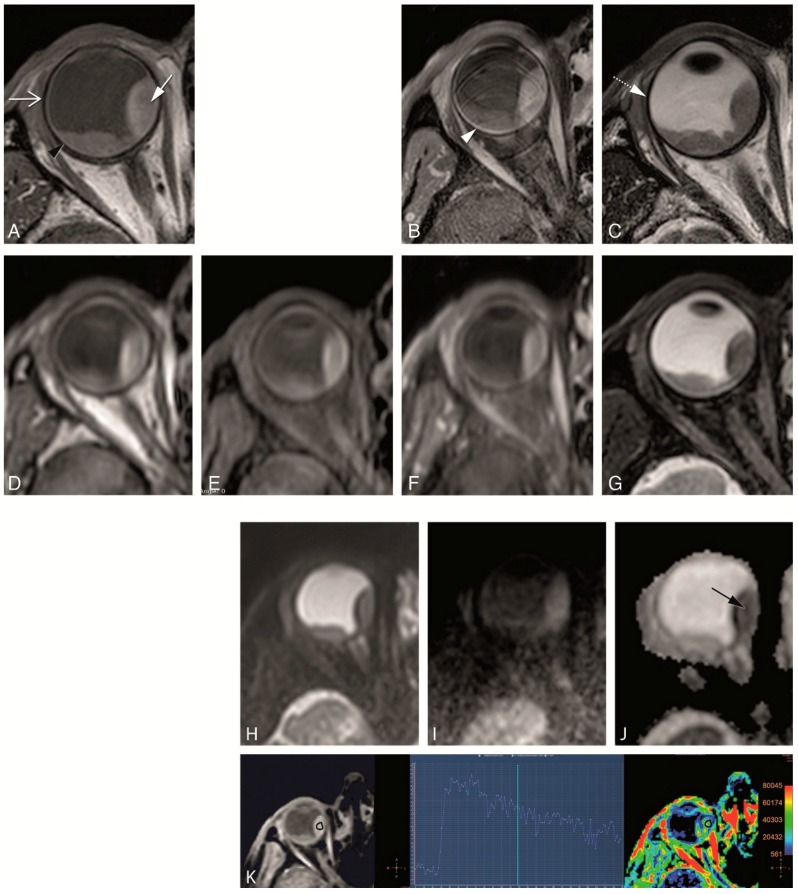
(A–K) Clinical protocol with anatomical and functional sequences in a patient with a uveal melanoma of the right eye (arrow). (A) MS 2 mm T1. (B) MS 2 mm enhanced T1 with fat signal suppression. (C) MS 2 mm T2. (D) 3D TSE 1 mm T1. (E) 3D TSE 1 mm T1 with fat signal suppression. (F) 3D TSE 1 mm enhanced T1 with fat signal suppression. (G) 3D TSE 0.8 mm T2 with fat signal suppression. (H–J) TSE DWI, with b values of 0 (H), 800 (I) and ADC (J). Restricted diffusion in the uveal melanoma (black arrow). (K) DCE with a good quality, few motion artefacts, showing a wash-out TIC pattern. Notice that on T2 WI the globe wall appears as a hypointense line (dashed arrow) and the different layers cannot be separated. On T1 the outer hypointense line corresponds to the sclera (open arrow), while the inner hyperintense layer corresponds to both the choroid and retina (black arrowhead). After contrast only the choroid and retina enhance (arrowhead). Anteriorly the ciliary body and iris can also be identified. The aqueous humor and vitreous body have a signal intensity similar to water. On the contrary, the lens is hypointense on T2 and slightly hyperintense on T1.
